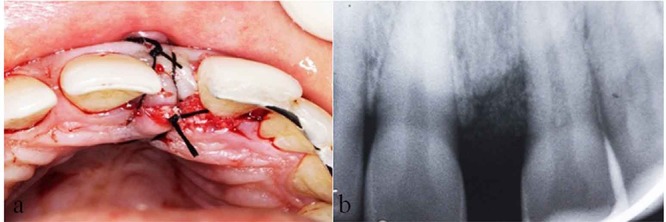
a: Flaps sutured with No.3-0 silk.  b: Post operative immediate radiographic view.
